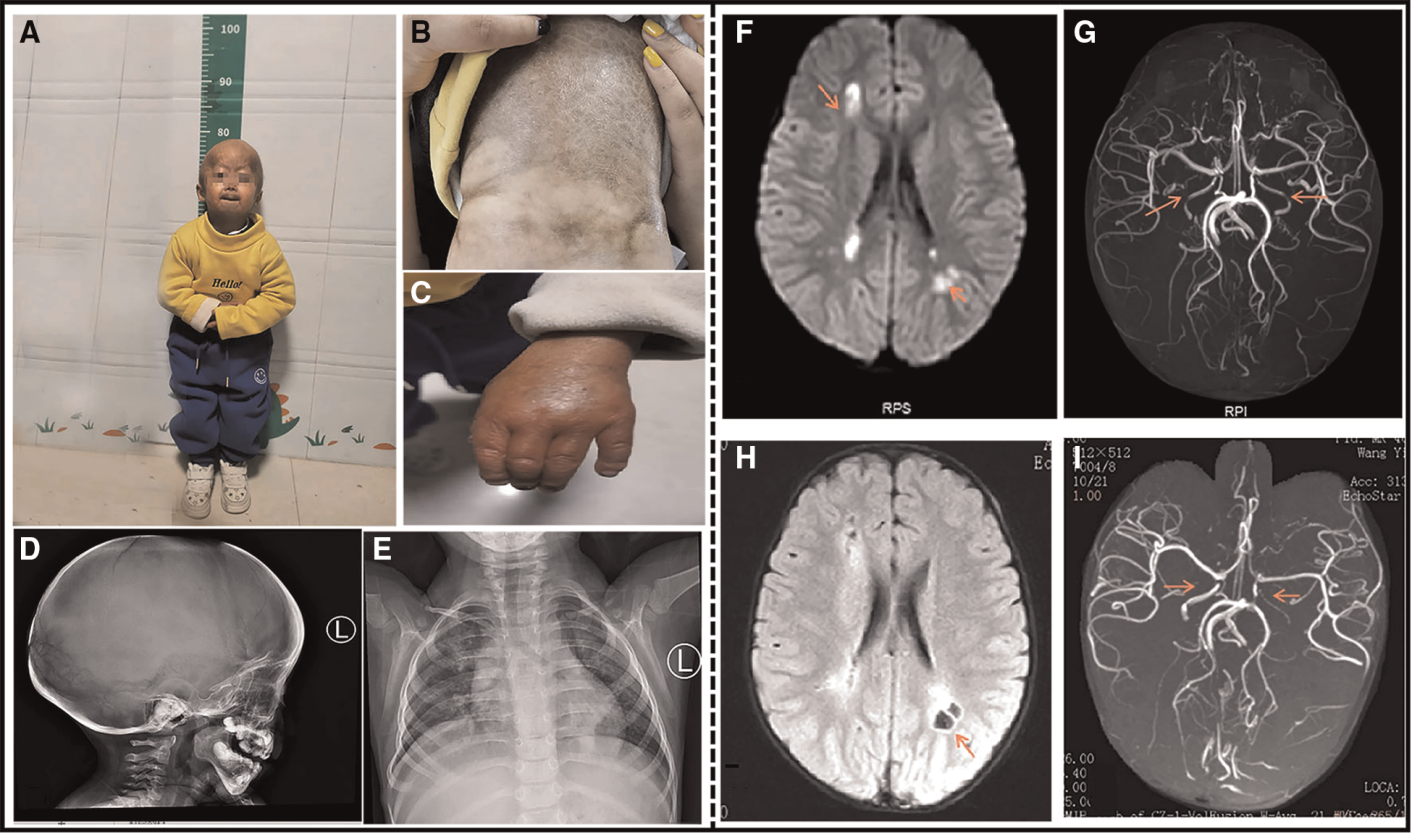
A 4-year-old boy patient. (A) The child has severe growth retardation; he showed distinctive facial features, scalp veins, generalized alopecia with sparse downy hairs, narrow nasal bridge, thin lips, and micrognathia. (B) Dry and stiff skin on the entire body, poor elasticity, and a lot of mottled pigmentation on the trunk. (C) Flexion deformity of the proximal phalangeal joints of both the fingers. (D) The shape of the head is enlarged, the anterior fontanelle and cranial suture are not closed, and the cranial plate of the parietal occipital region is thinned. (E) Multiple deformities of the ribs on both sides, dysplasia of the clavicle and scapula on both sides. (F) Cranial MRI at the time of onset: Multiple abnormal signals around the lateral ventricle. (G) Cranial MRA at the time of onset: The red arrow indicates bilateral internal carotid artery stenosis. (H) After the 3-month review, Cranial MRI: bilateral paraventricular lacunar infarction. (I) After the 3-month review, Cranial MRA: The red arrow indicates bilateral internal carotid artery stenosis similar to that noted earlier.
